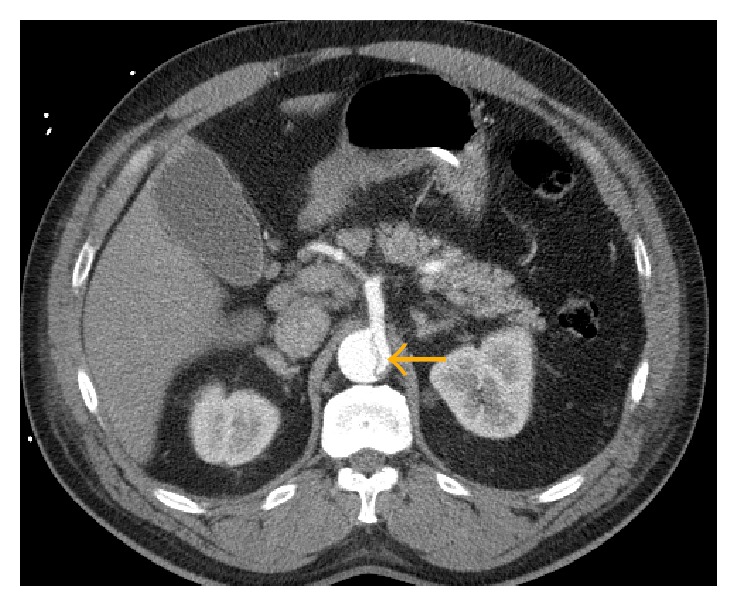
CT angiography showing acute type B aortic dissection with severe narrowing of the true lumen (yellow arrow).
